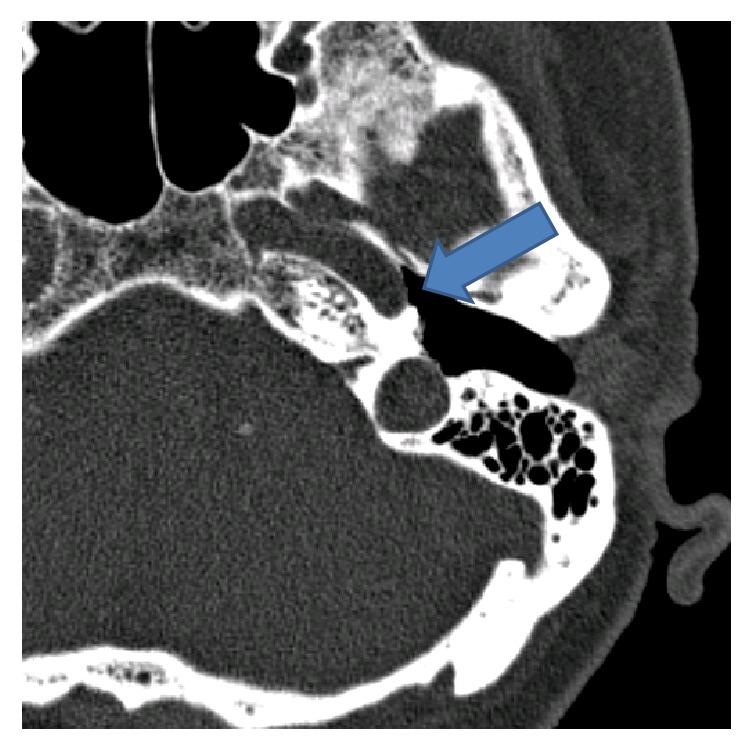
CT scan of a possible bony dehiscence of the internal carotid artery. Arrow indicates dehiscence.
